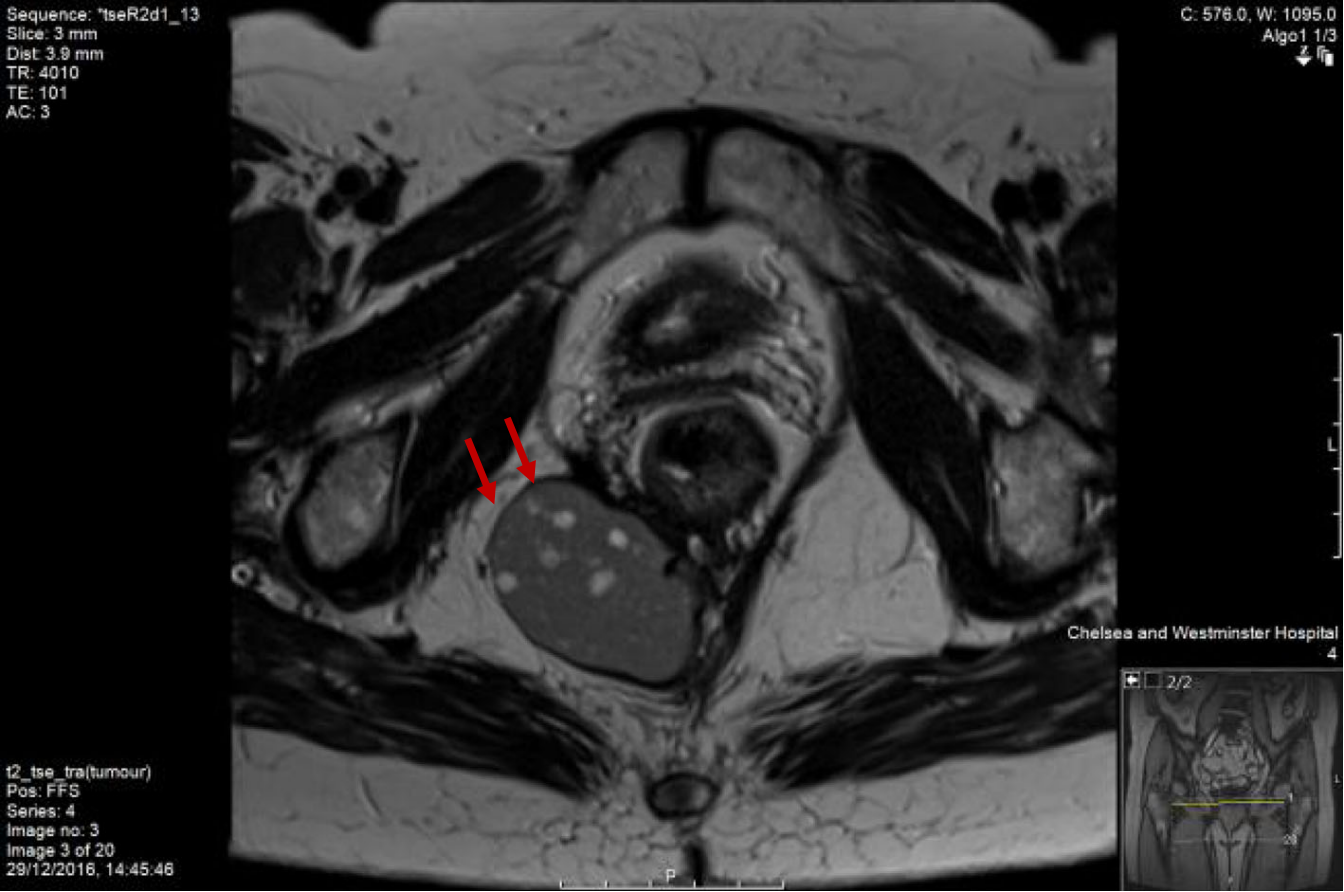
MRI scan image—transverse view
